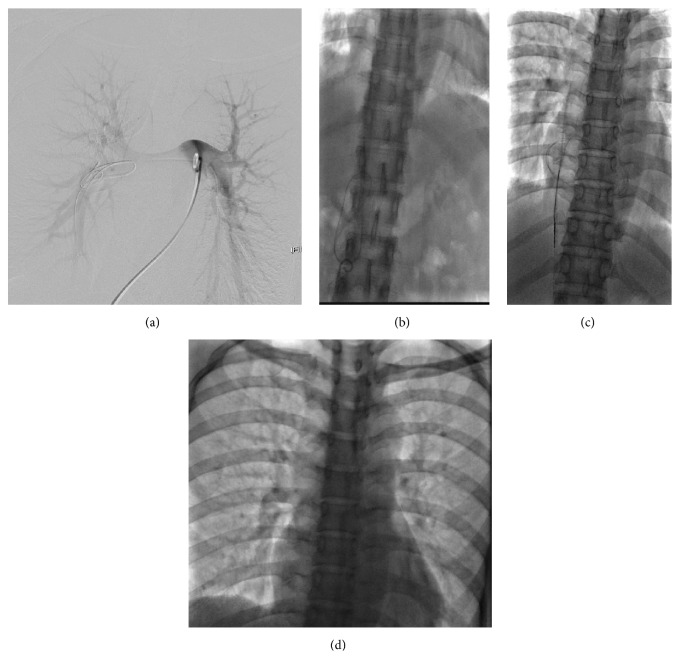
(a) The proximal and distal ends of the fractured catheter were located in the left and right branches of the pulmonary artery trunk, respectively, and the fractured catheter body was grasped by a pigtail catheter. (b) The fractured catheter was brought to the lower segment of the inferior vena cava to be removed. (c) The fractured catheter was grasped by the loop system of the vena cava filter retrieval set to be removed. (d) Chest radiograph after percutaneous retrieval of the fractured catheter, demonstrating the absence of any residual fragments.
